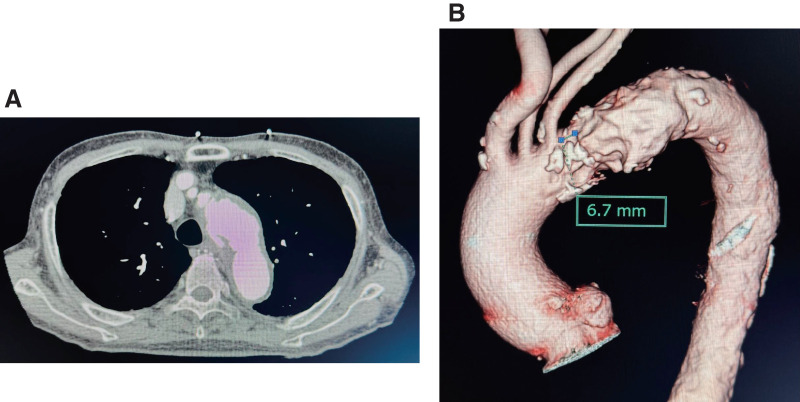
(A) Preoperative contrast-enhanced CT (axial view) showing a large ulcer-like projection just distal to the left subclavian artery. (B) Preoperative 3D CT indicating a proximal landing zone of 6.7 mm. 3D: 3-dimensional; CT: computed tomography
